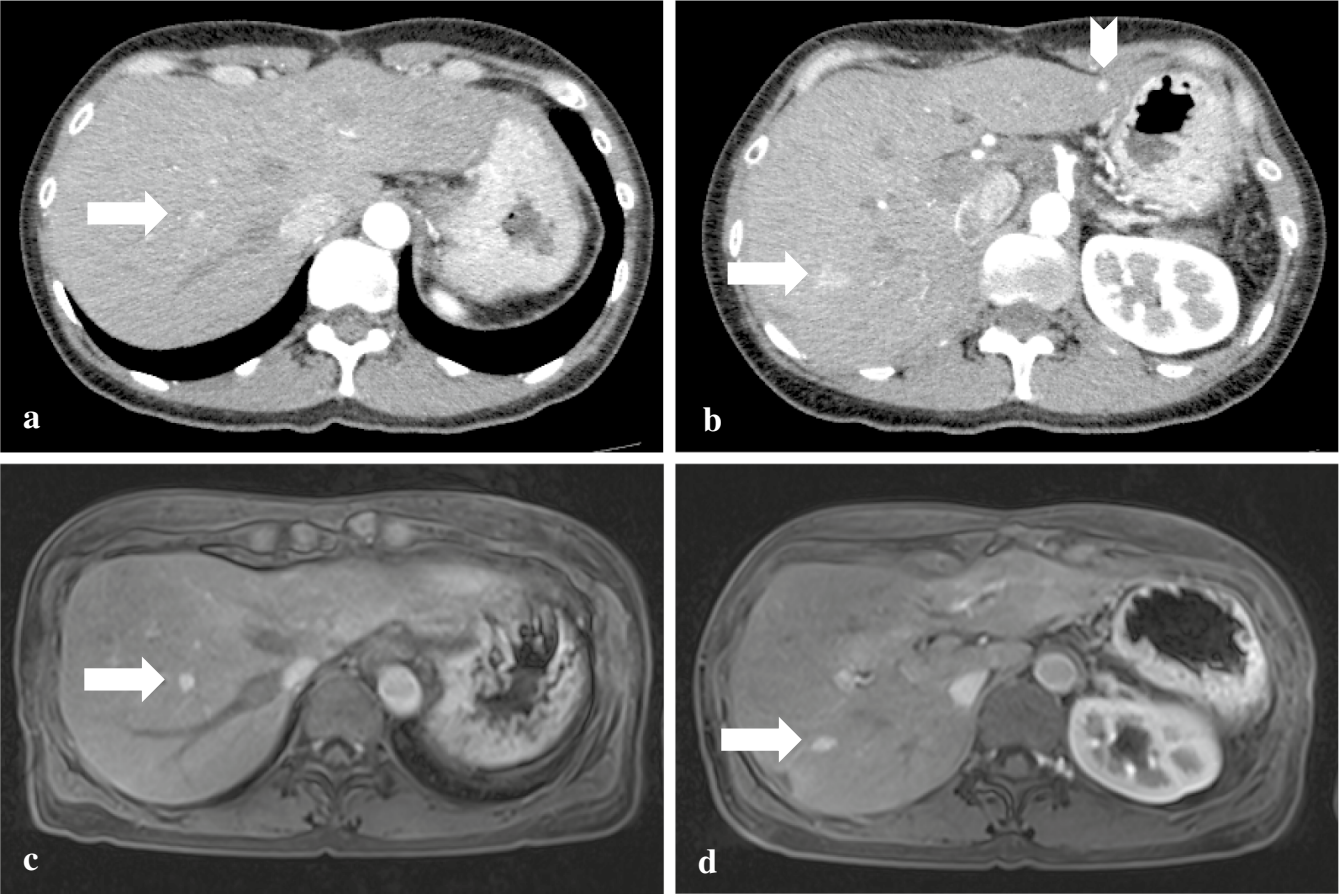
a, b Contrast-enhanced CT scan. Post-arterially hyper-enhanced lesions were revealed in the liver segment VIII (a, arrow), segment III (b, arrowhead), and VIII (b, arrow). c, d Gd-EOB-DTPA-enhanced MRI. Two arterially hyper-enhanced lesions were revealed in liver segment VIII (c and d, arrow). The lesion of segment III detected by the CT scan was undetectable. *CT* computed tomography, *Gd-EOB-DTPA* gadolinium ethoxybenzyl diethylenetriamine penta-acetic acid, *MRI* magnetic resonance imaging
